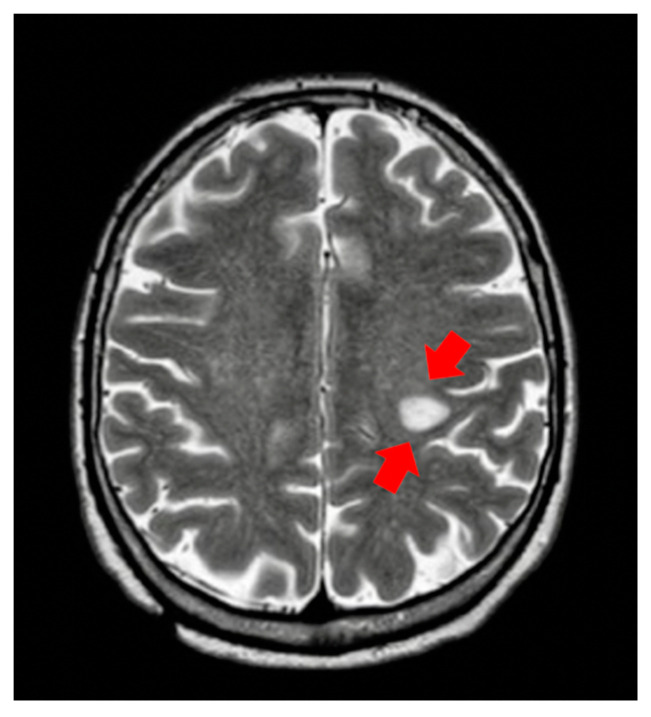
First brain magnetic resonance imaging showing encephalic vasculitis during sunitinib treatment.
